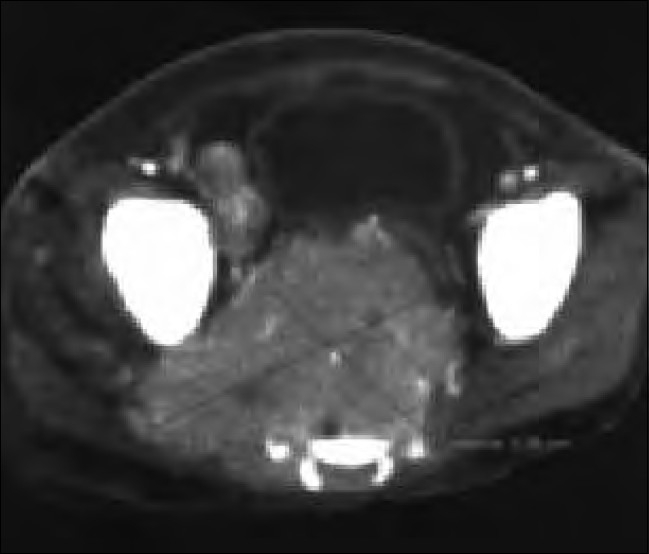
Patient 3: Computerized tomography (CT) of the abdomen and pelvis. Contrast-enhanced axial CT image through the lower pelvis. Heterogeneously enhancing soft tissue mass destroying the sacrum. It displaces the urinary bladder anteriorly and also extends into the right side. Another large lymphnode mass is also noted adjacent to the iliac vessels
